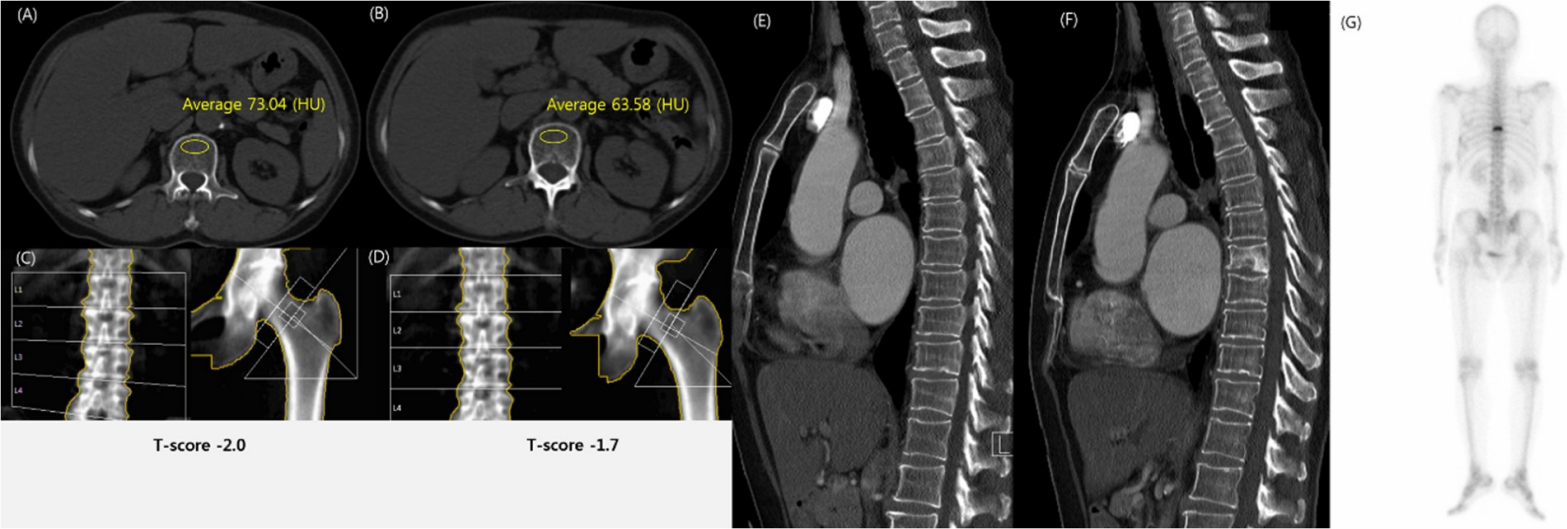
Compression fracture in T8 vertebral body in a 61-year-old woman with breast cancer. (A-B) Chest computed tomography (CT) scan showed a markedly decreased L1 trabecular attenuation in 2013 (73 HU, A) and 2014 (64 HU, B). (C-D) Dual-energy X-ray absorptiometry (DXA) was interpreted as osteopenia with a T-score of -2.0 in 2013 (C) and a T-score of -1.7 in 2014 (D). (E-G) Chest CT scan showed no fracture in 2013 (E). Sagittal CT scan (F) in 2014 and bone scan (G) showed a compression fracture in the T8 vertebral body. The diagnosis discrepancy between DXA and CT in this case suggests that DXA was falsely negative given the subsequently-identified fracture.
